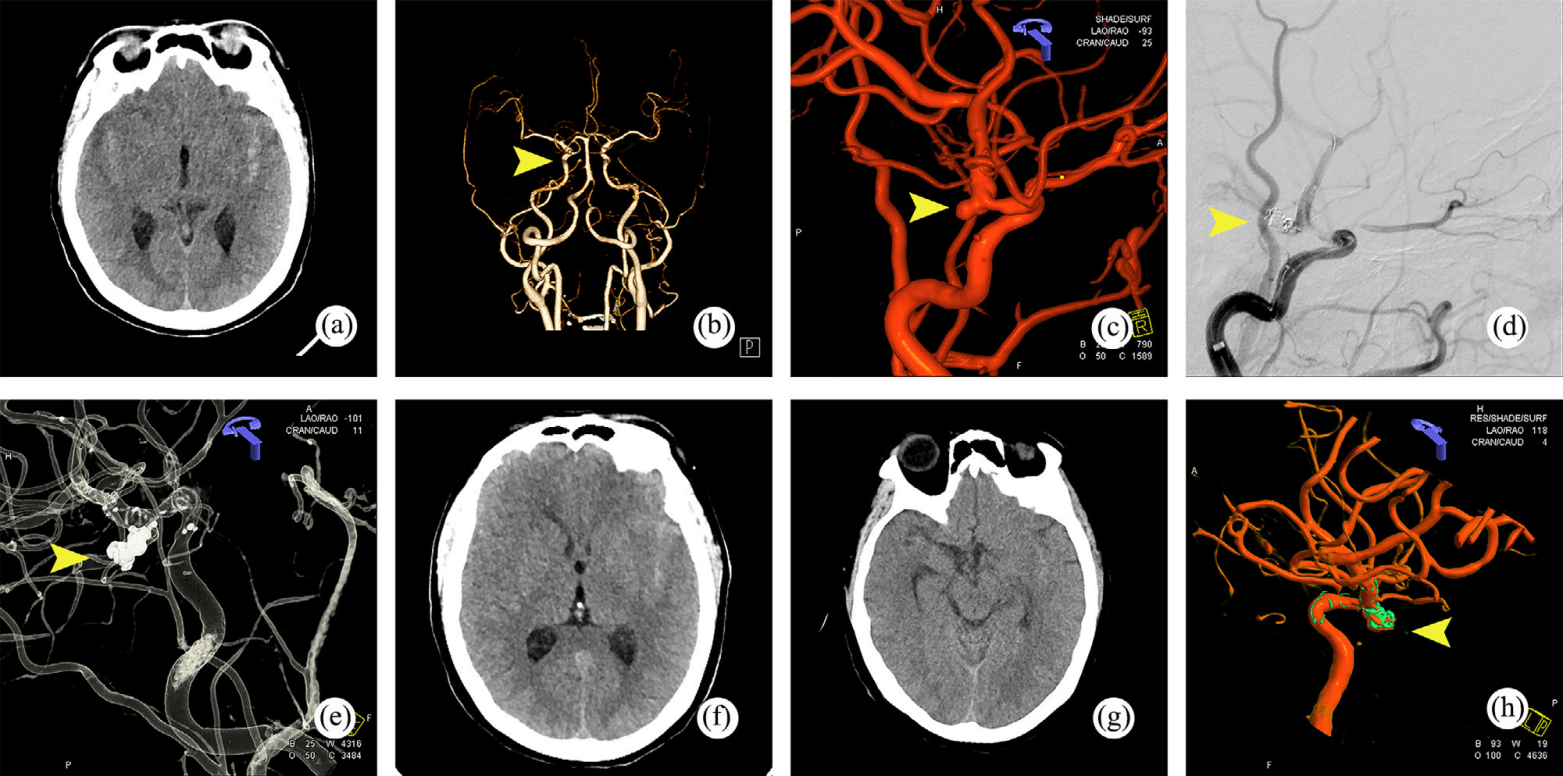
Representative case of subarachnoid hemorrhage due to a ruptured aneurysm on the C7 segment of left ICA. (a,b) Subarachnoid hemorrhage due to a ruptured aneurysm on the C7 segment of the left ICA on brain CTA. (c) Left ICA aneurysm identified by cerebral angiography with 3D reconstruction. (d) During embolization, contrast medium leakage was observed, possibly due to bleeding following aneurysm rupture. (e) Continued embolization leading to dense embolism. (f) Brain CT scan on the day of the surgery did not reveal any additional bleeding. (g) The bleeding was almost completely absorbed 8 days after the operation. (h) No evidence of in‐stent stenosis was observed during the cerebral angiography 3D imaging review conducted 8 months after surgery. ICA, internal carotid artery; CTA, computed tomography angiography.
